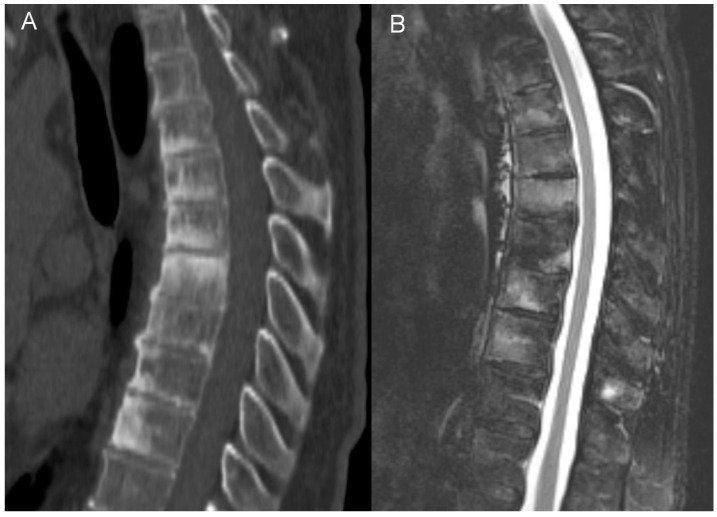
Imaging findings of the thoracic spine involvement in a 65-year-old patient with SAPHO syndrome. CT (A) shows multilevel, contiguous vertebral osteosclerosis, endplate erosions, and early syndesmophyte formation. MRI (B) shows diffuse and propagating patterns of BME and mild prevertebral soft tissue inflammation.
